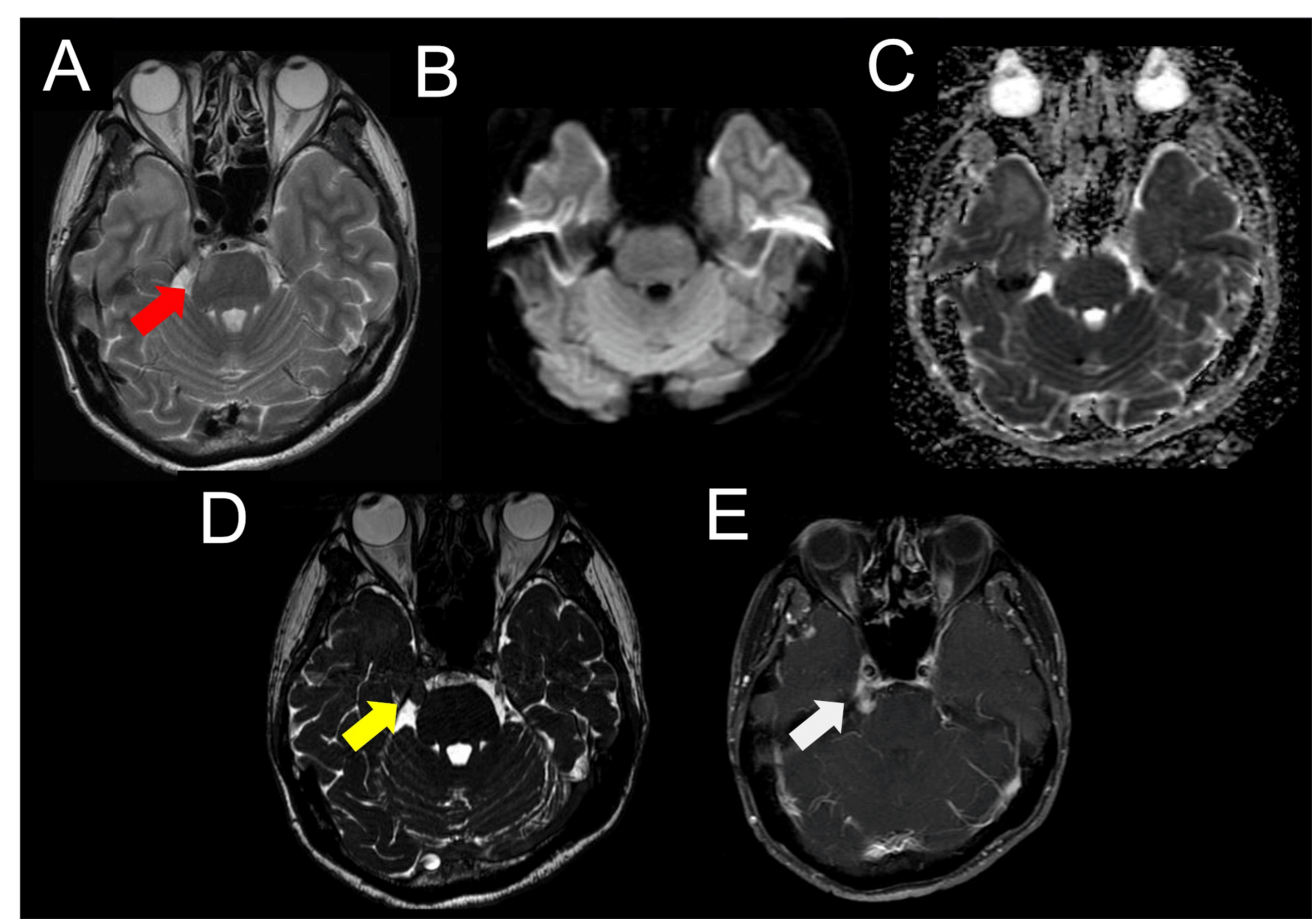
Brain magnetic resonance imaging was performed 15 days after onset. T2-weighted imaging revealed hyperintensity in the white matter of the right temporal lobe and the right pons (A: red arrow). The lesion in the right pons had an iso intensity on diffusion-weighted imaging (B) and elevated apparent diffusion coefficient values (C). An enlarged and contrast-enhanced right trigeminal nerve was observed on fast imaging employing steady-state acquisition sequences (D: yellow arrow) and contrast-enhanced T1-weighted sequences (E: white arrow), respectively. No contrast enhancement was observed in the pontine lesion.
